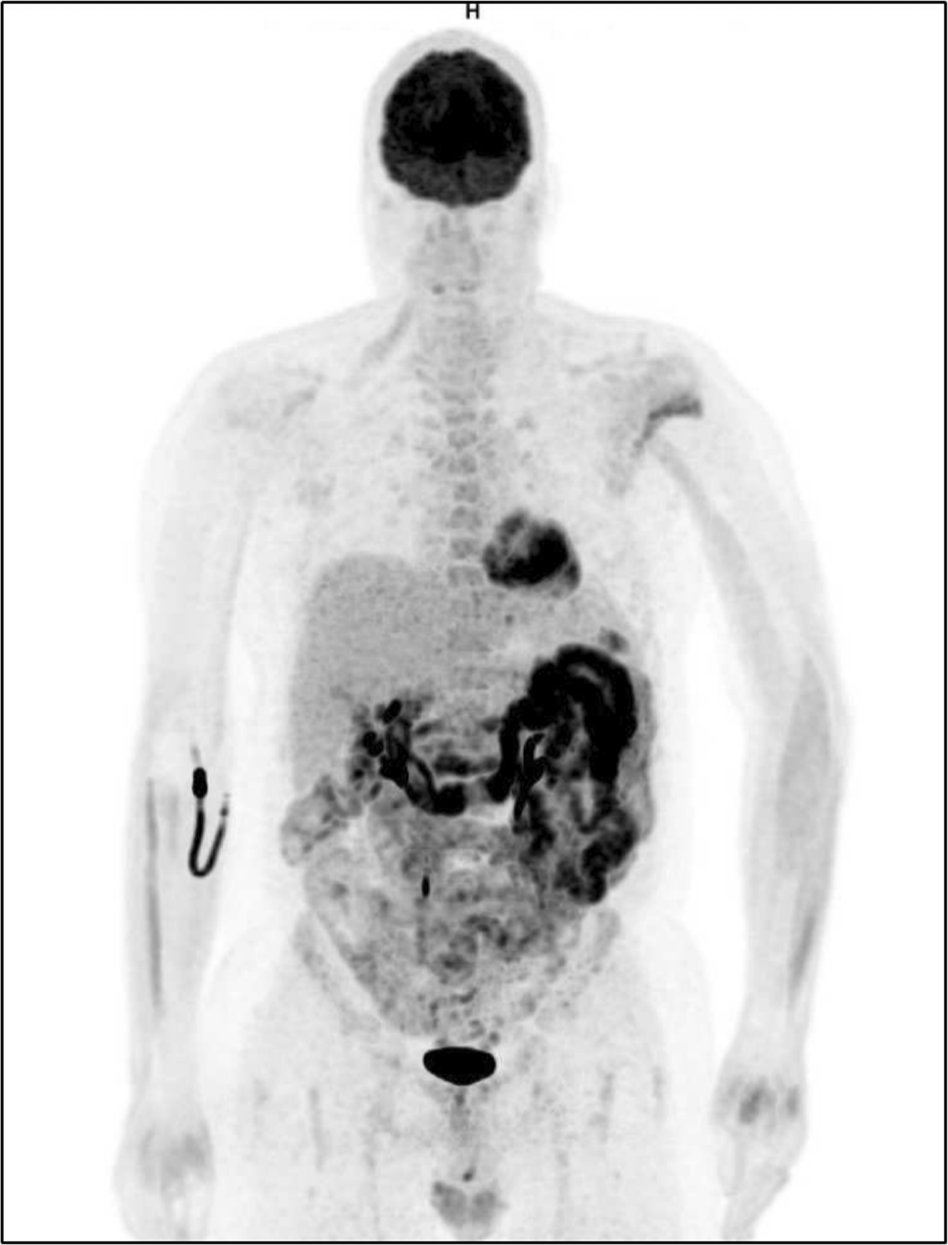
^18^F-FDG PET/CT image of a stage IV enterocolitis during anti-CTLA-4 treatment in a patient with metastatic melanoma. Immunotherapy was interrupted, and a high-dose steroid therapy was started
